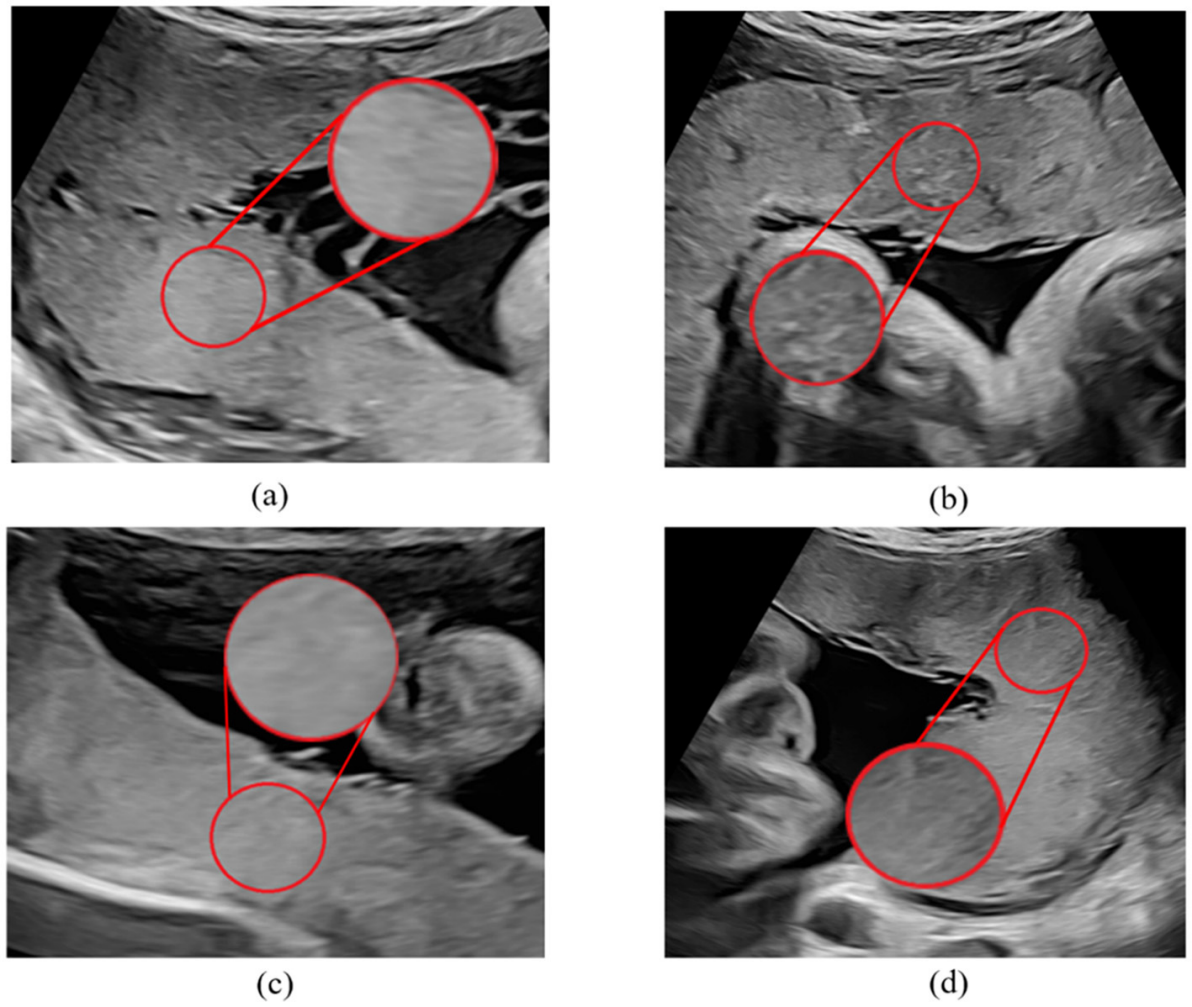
Sample of placental ultrasound images. (a, c) Are placental ultrasound images with FGR, and (b, d) are normal placental ultrasound images.
